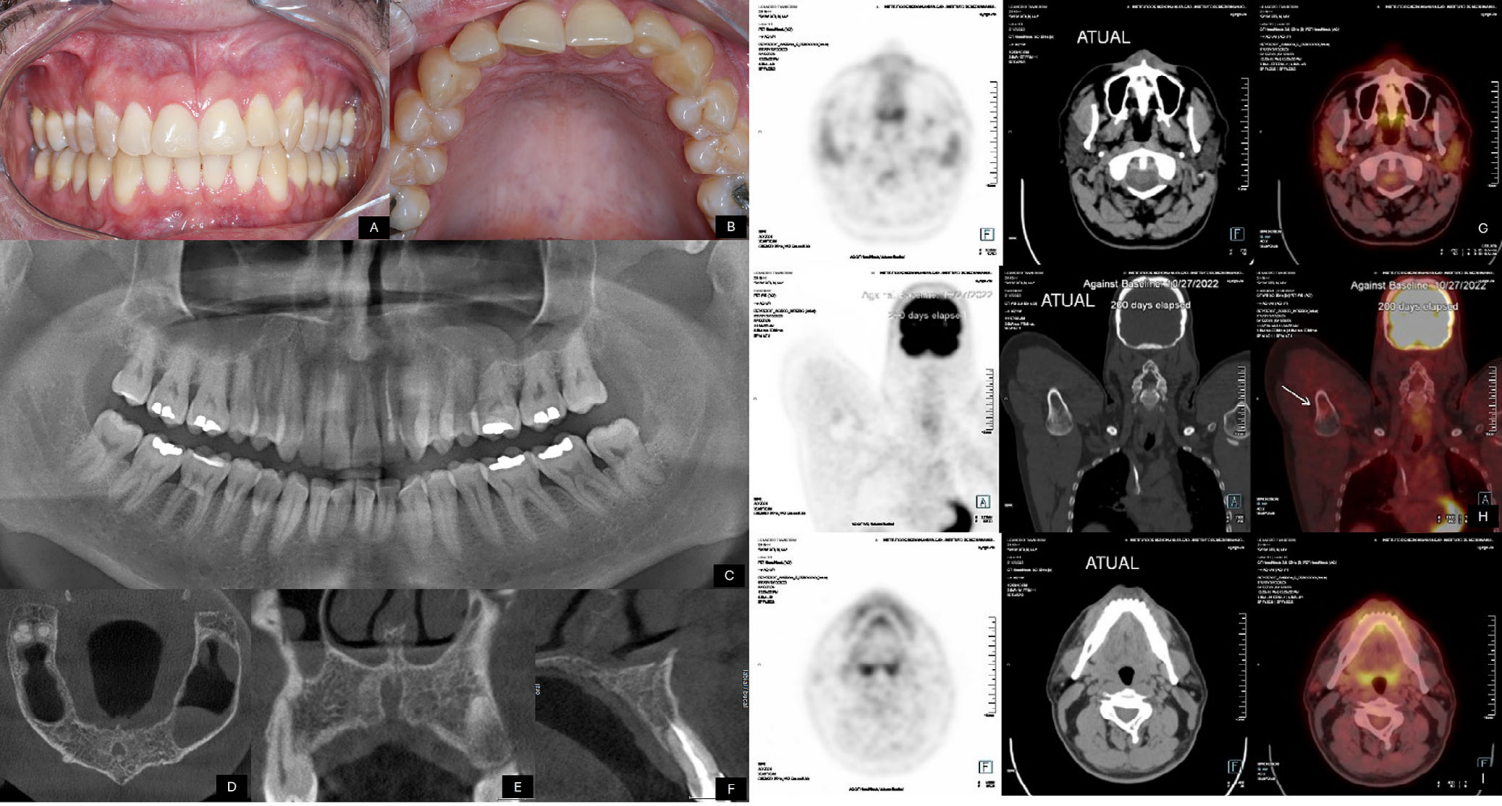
Clinical and radiographic assessment 18 months post‐treatment. (A‐B) Intraoral examination revealed intact, normochromatic oral mucosa, with no signs of swelling. The gingival “black space” in the interproximal region between central and lateral incisors had been successfully restored. (C) Panoramic radiograph demonstrating complete bone neoformation in the maxilla. (D‐F) Cone‐beam computed tomography (CBCT) scan confirming new bone formation in the anterior region of the left maxilla. (G‐I) PET/CT scan showing complete disease remission, with no evidence of hypermetabolic lesions in the maxilla, humerus, or cervical lymph node.
